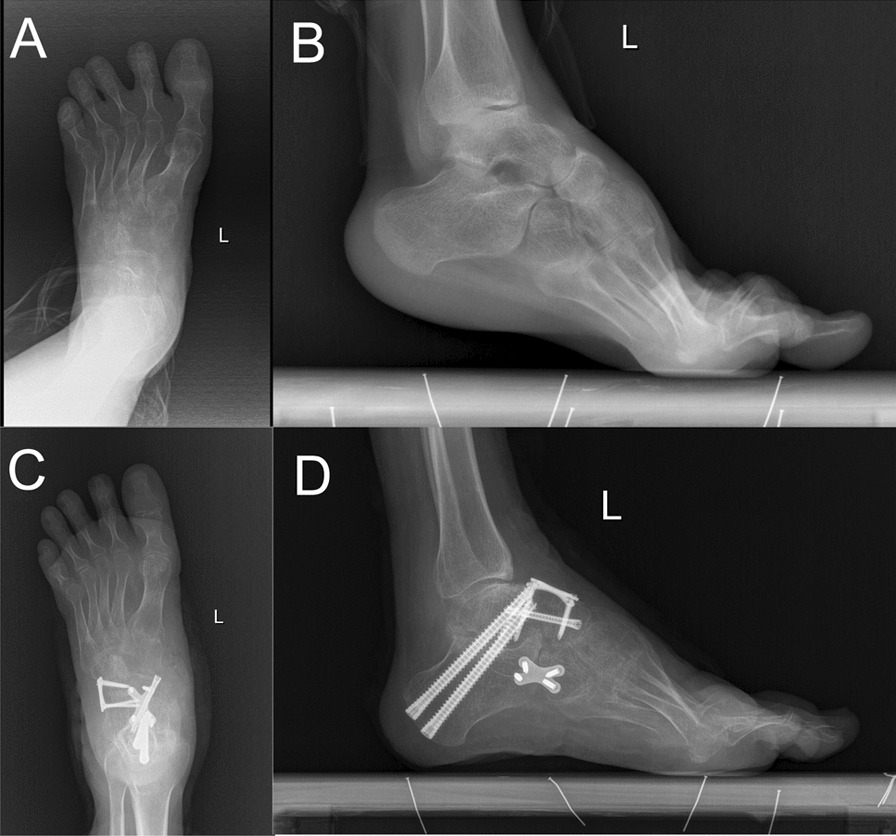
Results of pre- and post-operative X-rays. a Preoperative anterior–posterior foot X-ray. b Preoperative lateral foot X-ray. c Postoperative anterior–posterior foot X-ray. d Postoperative lateral foot X-ray
